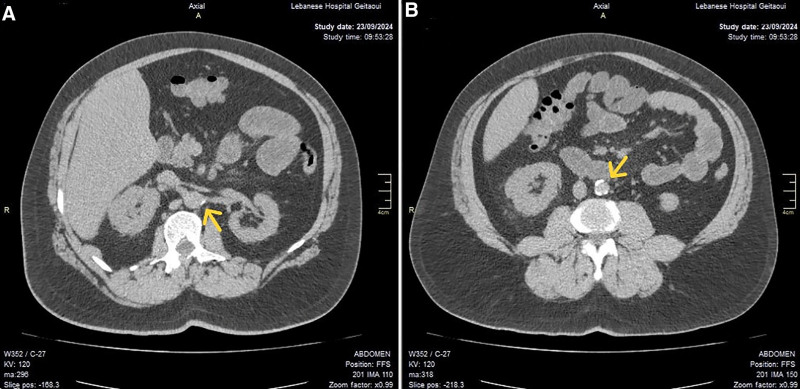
CT of the abdomen and pelvis without IV contrast showing calcifications on the origin of the left main renal artery (A) and the abdominal aorta (B).
